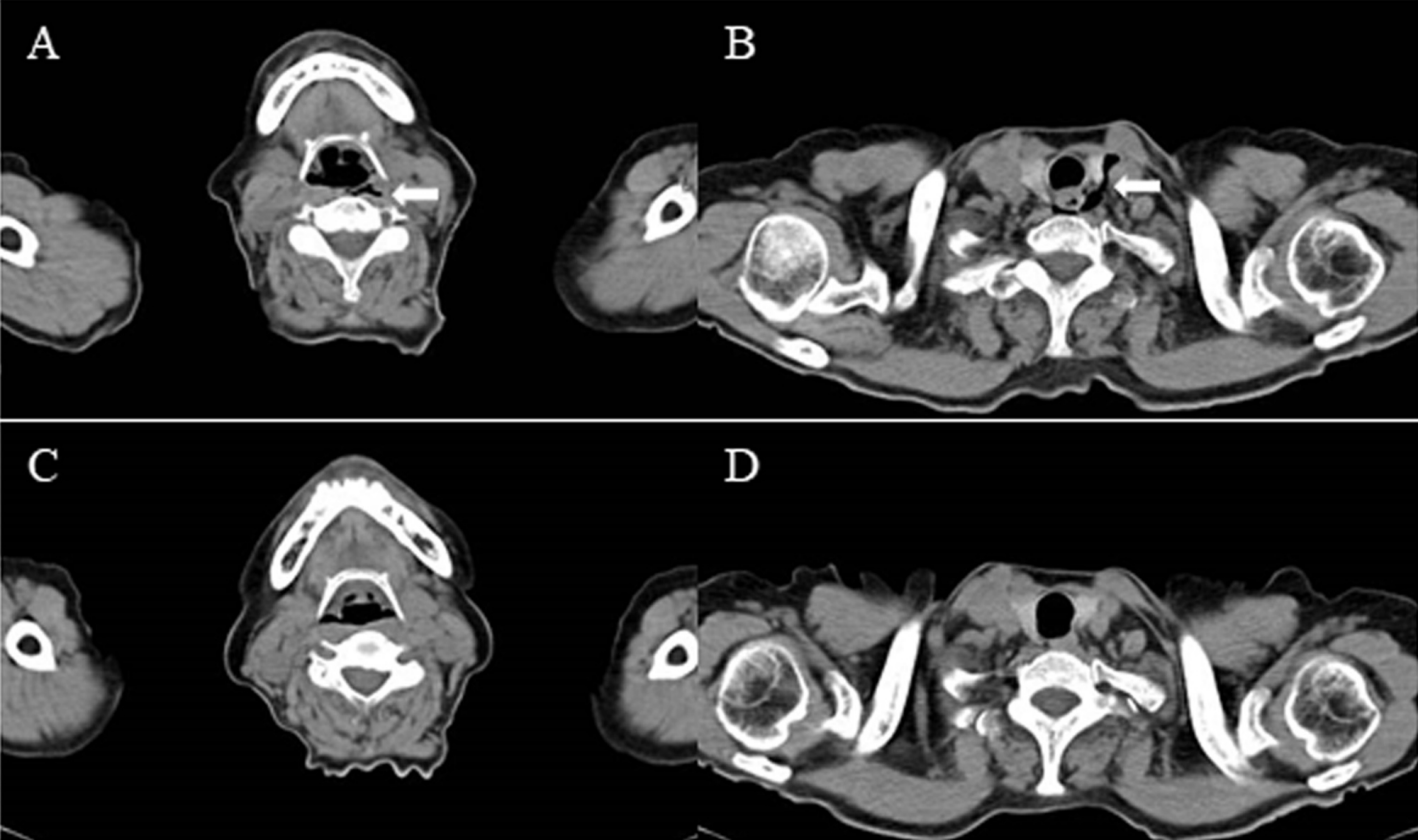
CT scan obtained immediately after ESD and follow-up CT scan obtained on postoperative day 5 CT scan obtained immediately after ESD (A) free air below the posterior wall of the oropharynx; (B) free air in the anterior mediastinum; (C) & (D) Follow-up CT scan obtained on postoperative day 5 showing the disappearance of the free air CT: computed tomography; ESD: endoscopic submucosal dissection
